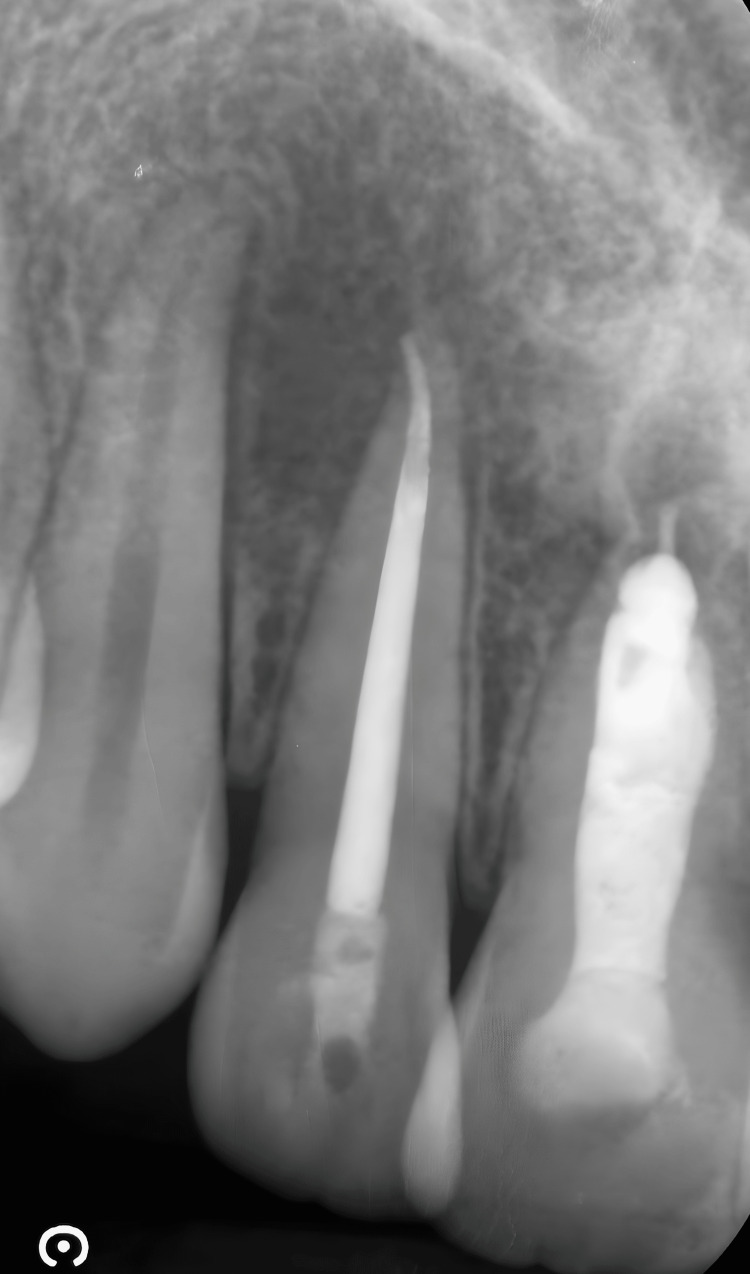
Intraoral X-ray at the six-month follow up visit showing the lesion's positive healing trend
